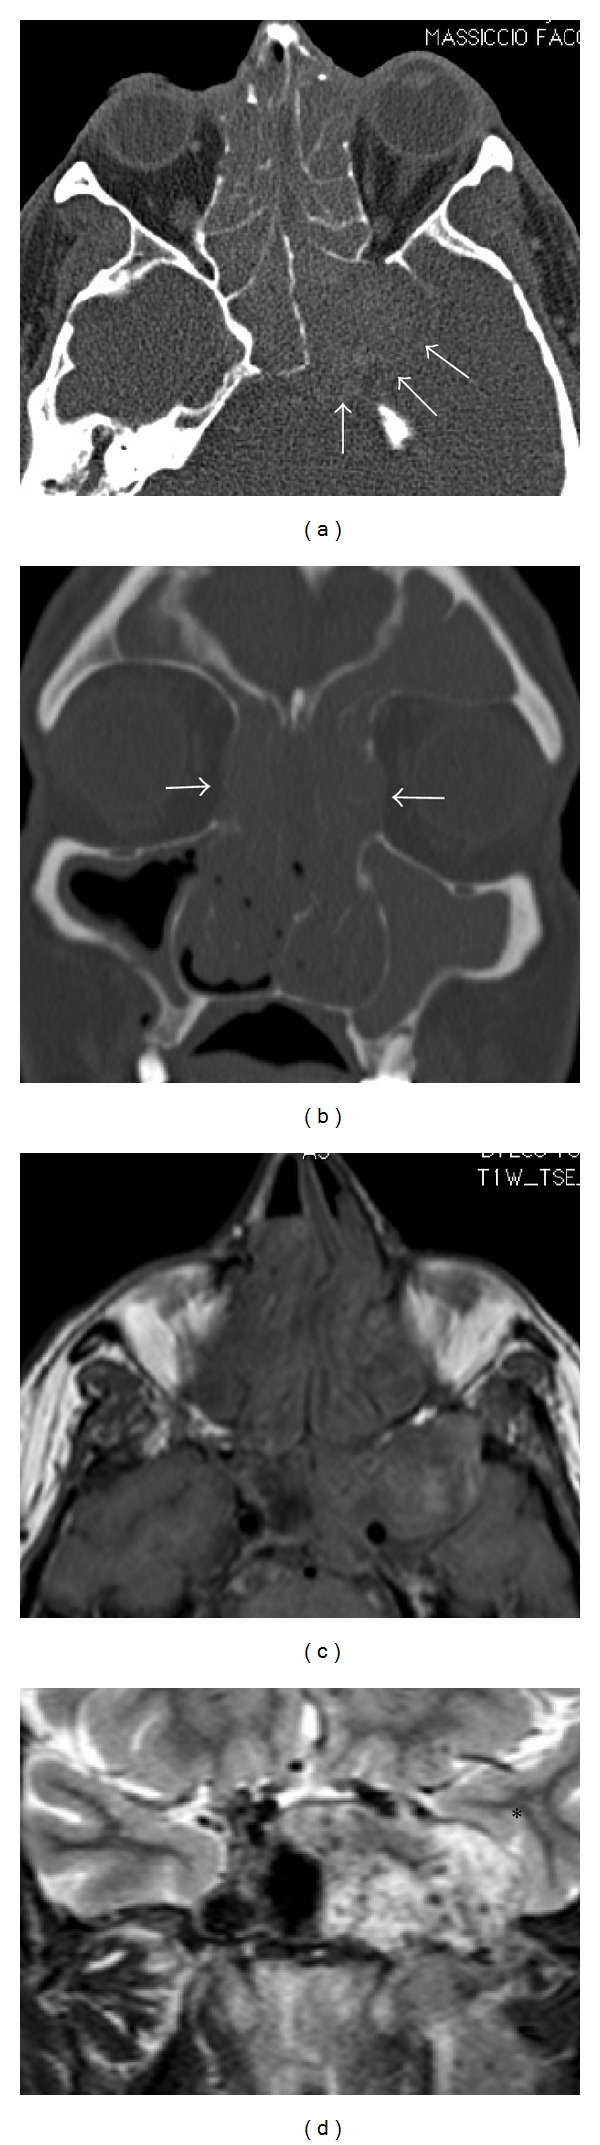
Maxillofacial and brain CT (a, b) and MRI (c, d). (a) Axial CT with contrast medium and (c) MRI-T1 weighted sequence showing abnormal extension of the left sphenoid sinus with thinning of the posterior and lateral bony walls (arrows) and compression of the temporal lobe (asterisk). (b) Coronal CT showing opacification of almost all paranasal sinuses associated with a focal erosion of the papyracea lamina (arrows). (d) Coronal T2-weighted MRI image showing temporal lobe compression (asterisk), while the left abducens nerve and cavernous sinus are not clearly recognizable.
